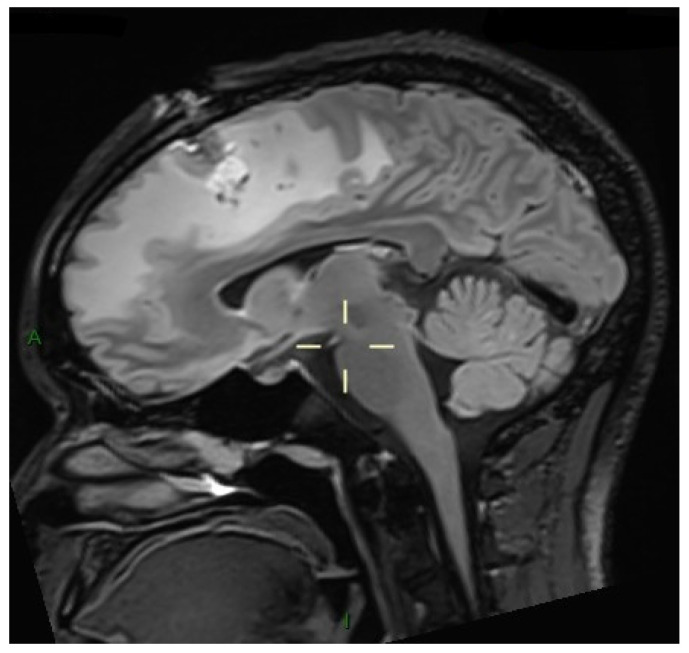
Axial MRI showing supratentorial ring-enhancing lesions with lobulated contours and surrounding vasogenic edema. Follow-up imaging demonstrates a marked reduction in lesion size, perilesional edema, and mass effect. The left frontal biopsy trajectory is also visible.
